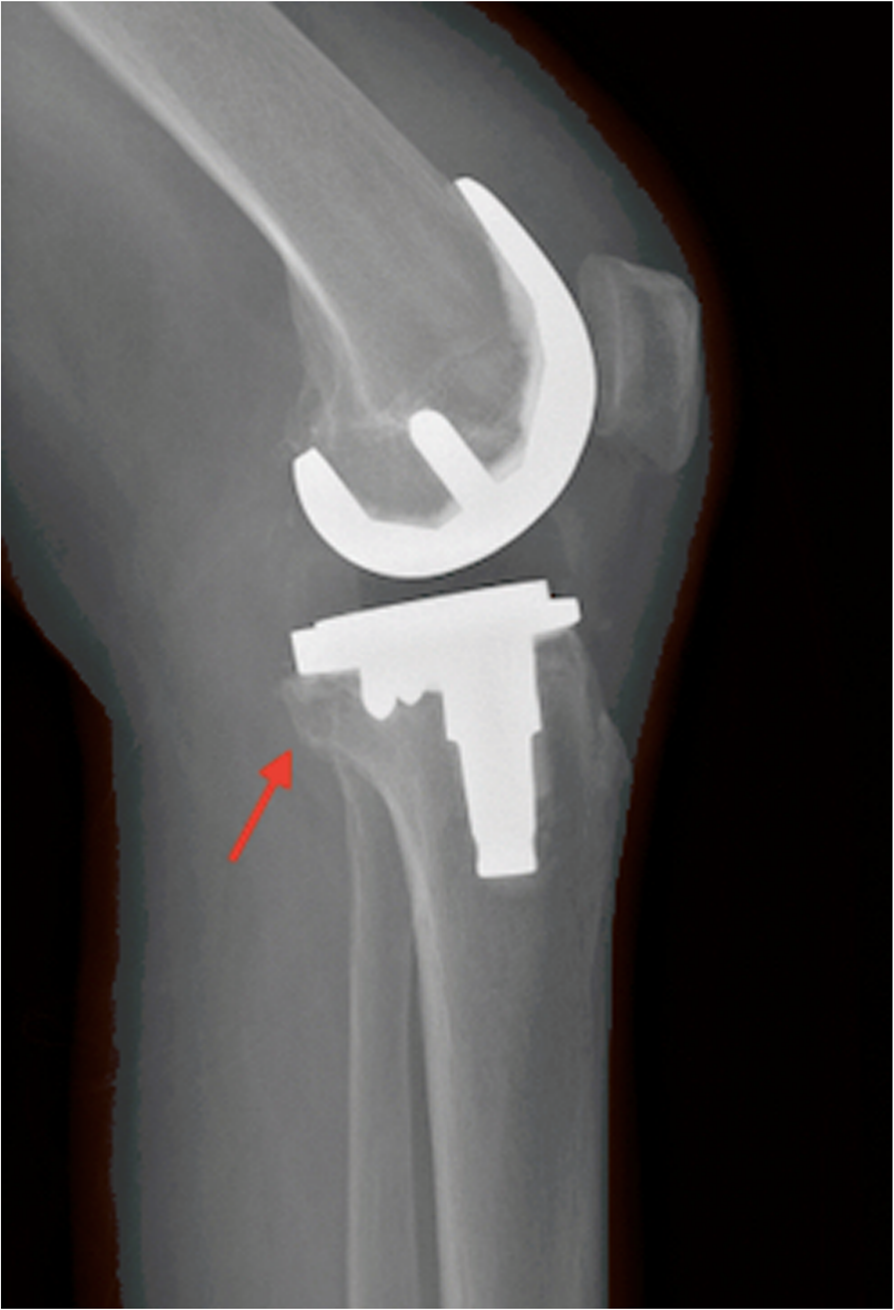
Lateral X-ray showing bone healing after 3 months
